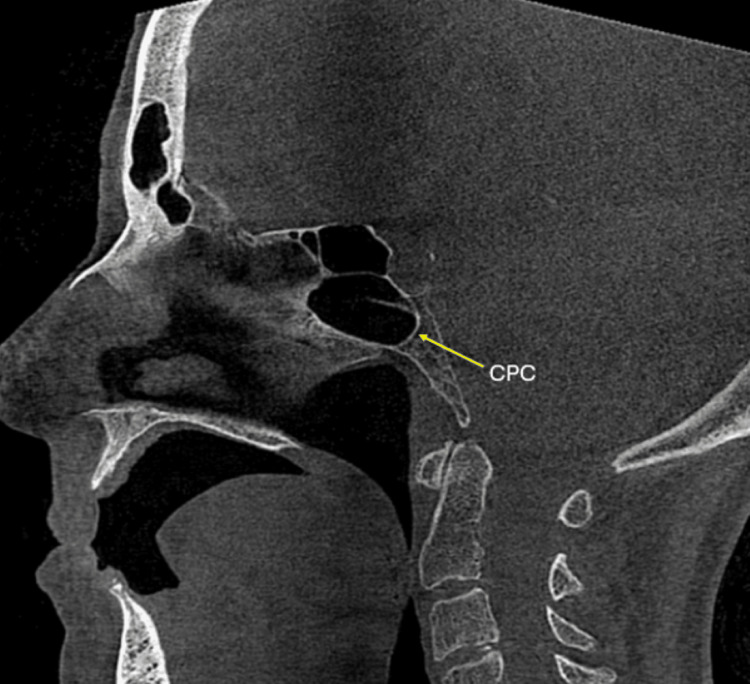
Sagittal CBCT section showing a persistent Craniopharyngeal Canal (CPC) as a corticated tubular channel at the sellar floor. CBCT, cone-beam computed tomography.
